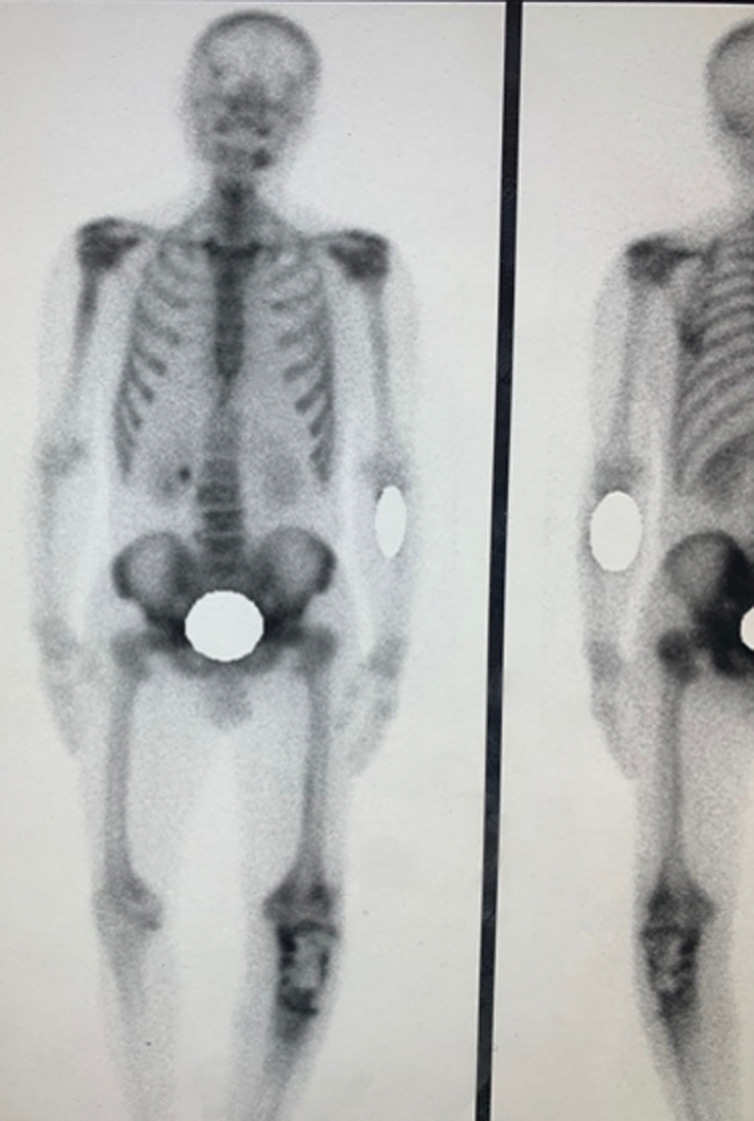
scintigraphie osseuse ; vue frontale (image de gauche) et vue dorsale (image de droite)
